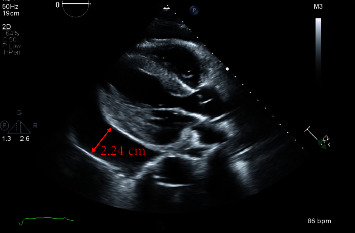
Initial transthoracic echocardiogram obtained on admission shows a large circumferential pericardial effusion measuring 2.24 cm (red line). Cardiac tamponade is present, evinced by early right ventricular diastolic collapse, significant respirophasic variation of mitral inflow and IVC dilation with decreased respiratory variation consistent with elevated right atrial pressure.
